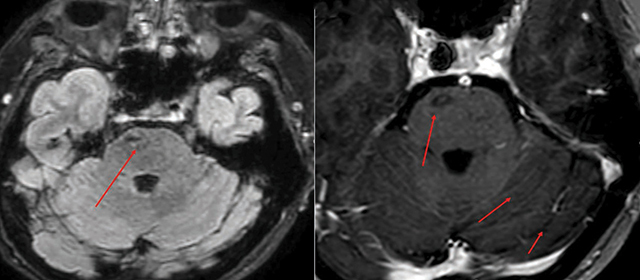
MRI images showing regression of edema in the right pontine region and persistent dilation of the cerebellar veins, indicating partial resolution of the condition.
